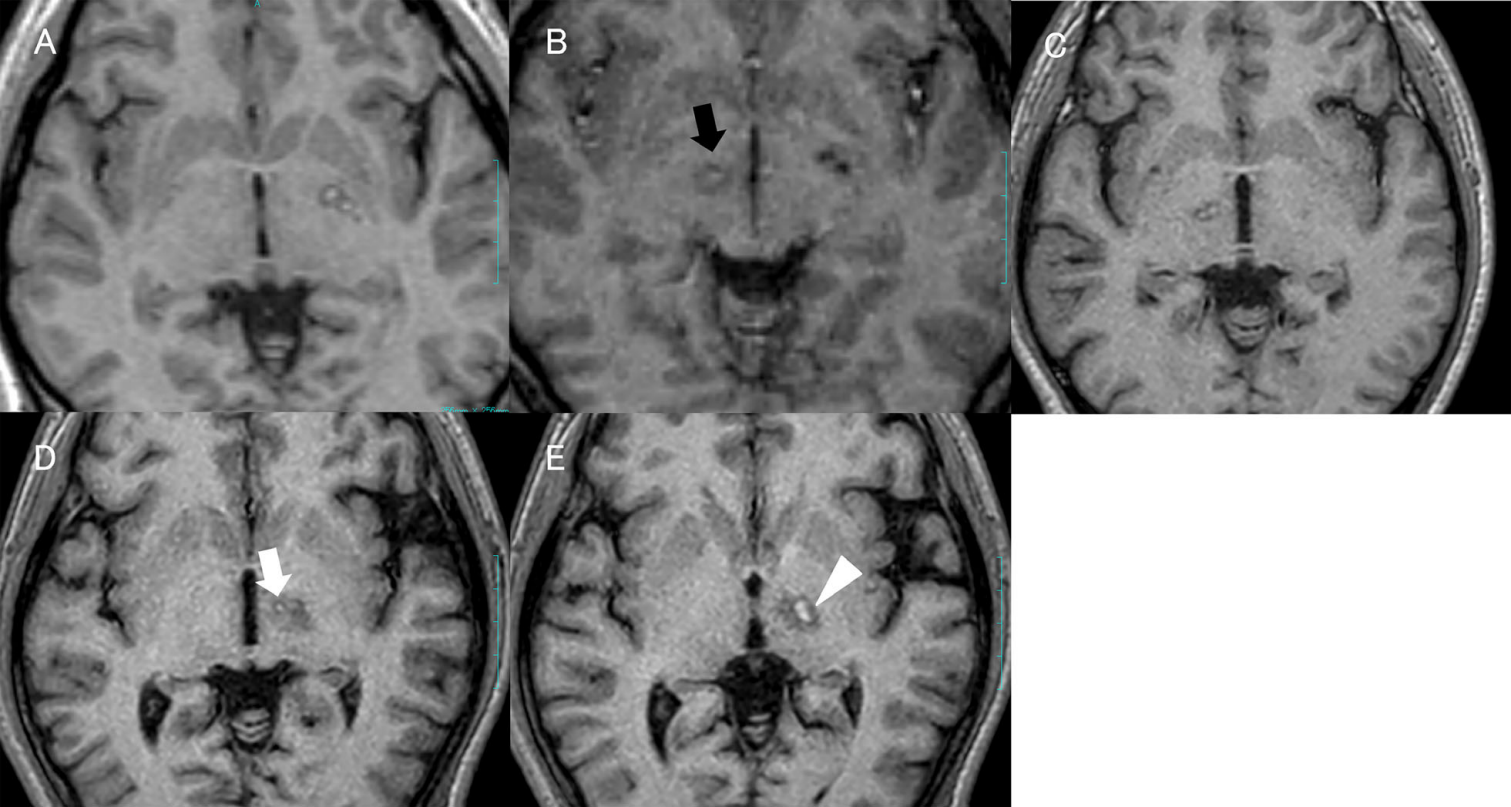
T1‐weighted magnetic resonance imaging on the day of surgery. Coagulated lesions on left globus pallidus internus (A) and right pallidothalamic tract (B, black arrow) of Patient No. 1, (C) Right pallidothalamic tract of Patient No. 2, (D) Left pallidothalamic tract (arrow) of Patient No. 3, and (E) Left ventro‐oral nucleus of the thalamus (arrowhead) of Patient No. 3.
